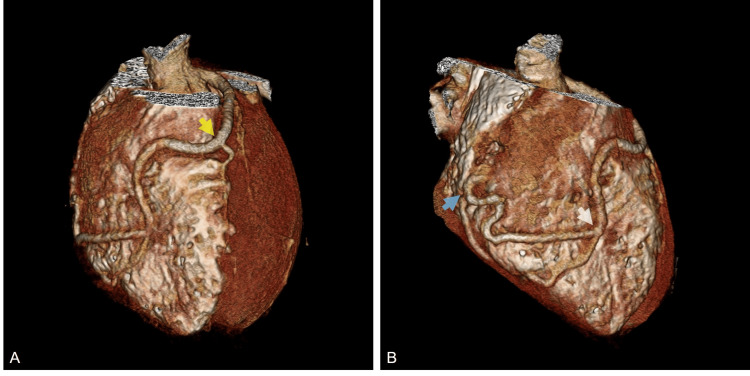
Computed tomography angiography three-dimensional images demonstrate fistula origin from the mid-left anterior descending artery (yellow arrow), which bifurcates into two branches (white arrow) and terminates near the base of the right ventricle outflow (blue arrow).
